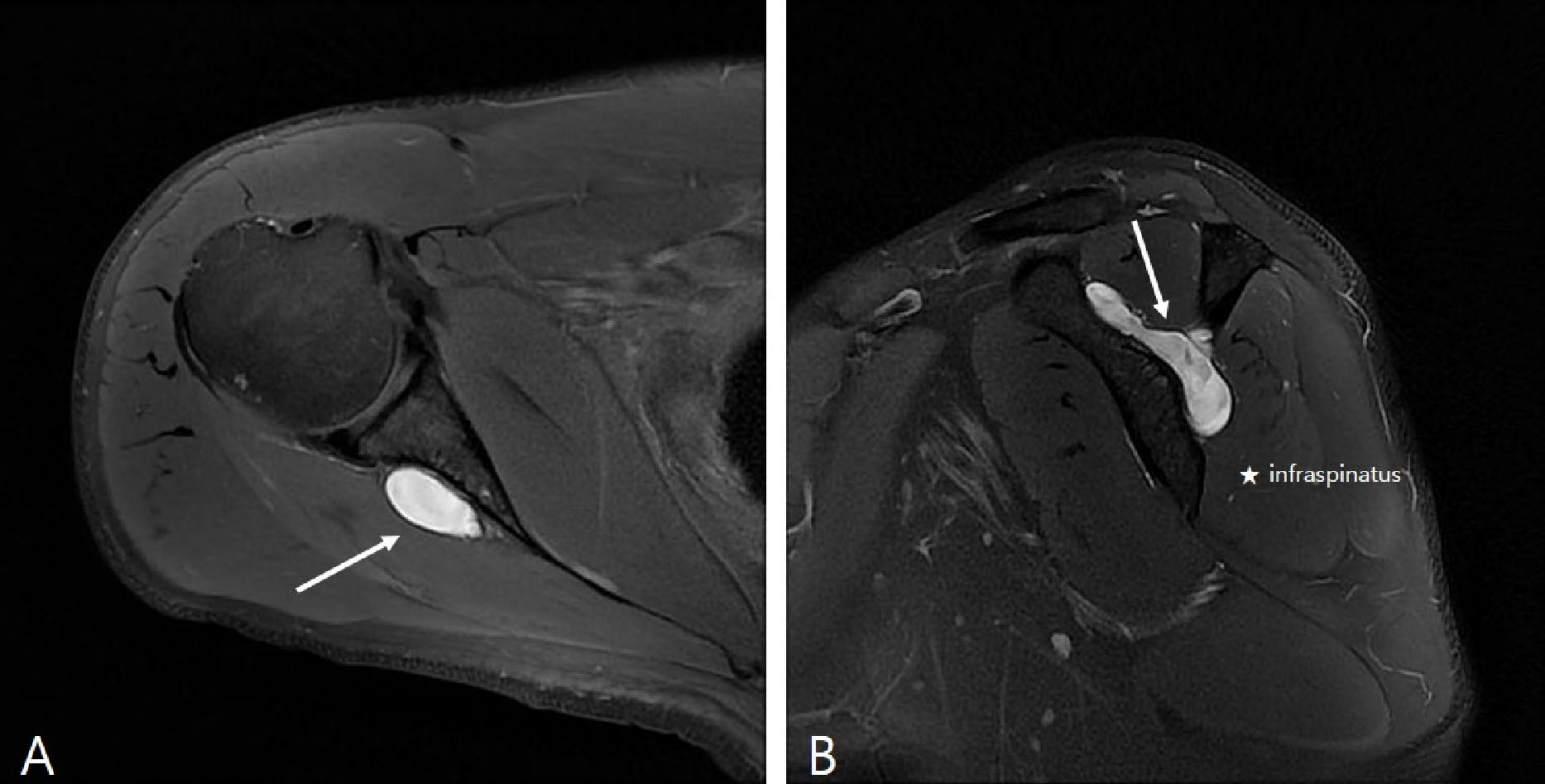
Preoperative magnetic resonance image of the lesion A The lesion (line arrow) exhibits high signal intensity on T2-weighted axial imaging. B Sagittal section revealing the heterogenous signal intensity of the cyst (line arrow) with the scapular spine of the right shoulder and infraspinatus muscle (star) denervation
